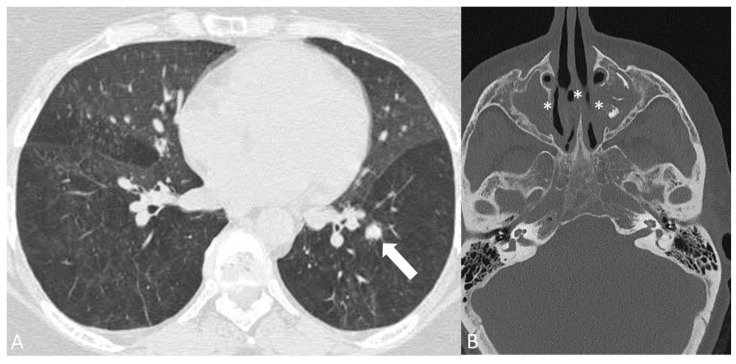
A 48-year-old man with granulomatosis with polyangiitis. CT chest without contrast (A) demonstrating a 1.5 cm solid non-calcified pulmonary nodule in the basal left lower lobe (arrow). CT of the facial bones without contrast (B) showing diffuse mucosal thickening with near complete opacification of the maxillary sinuses with erosion of the medial wall of the maxillary sinuses and nasal septum (asterisks).
